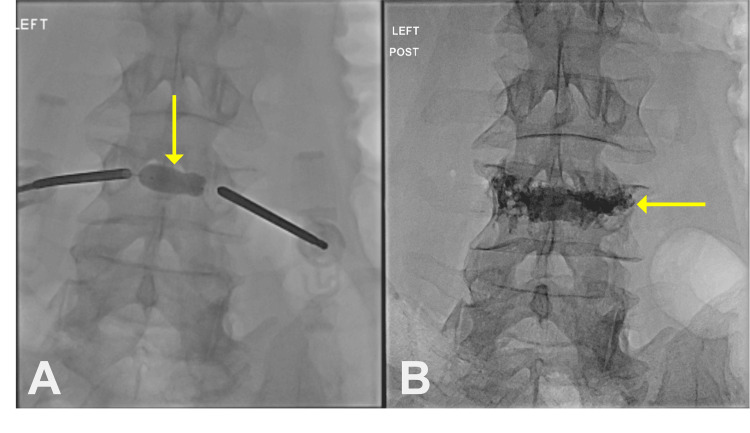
Computed tomography fluoroscopic imaging showing (A) the inflated balloon creating a cement cavity in the L4 vertebral body (yellow arrow) and (B) the L4 vertebral body filled with cement (yellow arrow)
